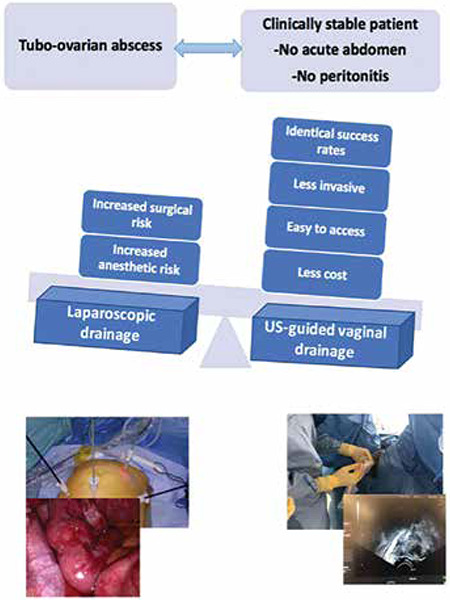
Graphical abstract. Comparison between laparoscopic and transvaginal approaches of adnexal abscesses US: Ultrasound
